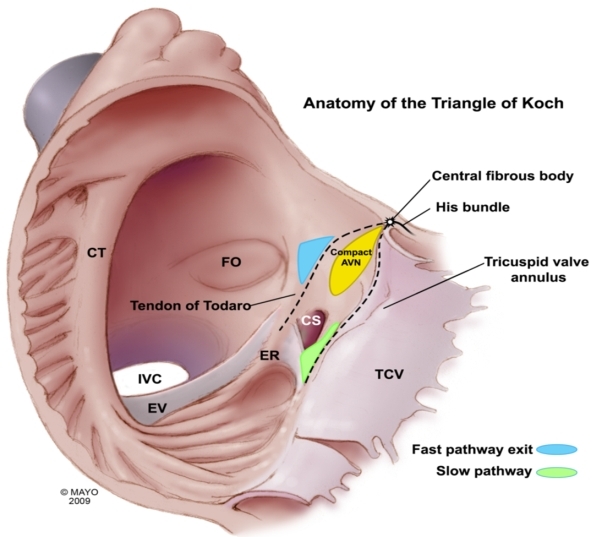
The true interatrial septum near the tricuspid annulus consists of the anterior and midseptal regions.  Midseptal pathways are inserted into the triangle of Koch region, close to the compact AV node.  Accurate assessment of pathway slant and ablation preferably of the ventricular insertion site along with the use of cryoenergy may all be required to minimize the risk of AV block when ablating pathways in this region. CT = cristoterminalis ; FO = Forman ovale; EV = Eustachian valve; IVC = inferior vena cava; TCV = tricuspid valve; CS = coronary sinus; ER = Eustachian Ridge; AVN = AV node
